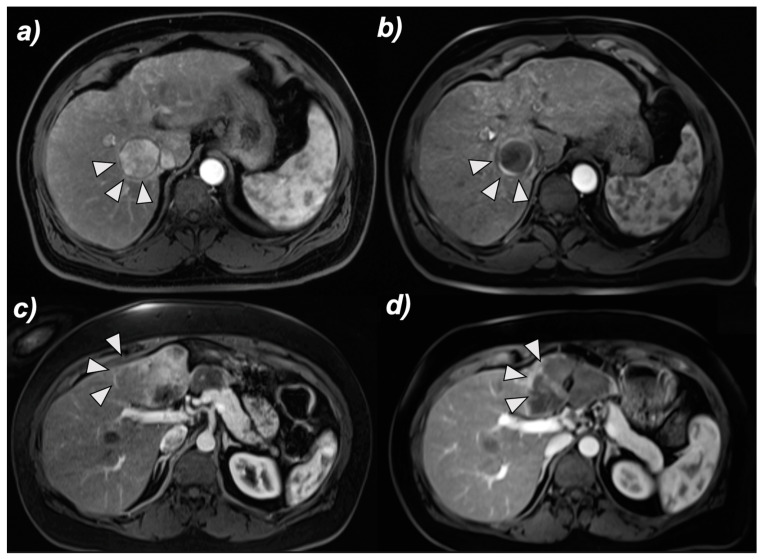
Imaging-based response of nodular and infiltrative HCC to TACE. Representative contrast-enhanced MR images of a nodular HCC treated (a) with DEB-TACE (upper row) and an infiltrative HCC (c) treated with cTACE (lower row), both showing good local tumor response on follow-up MRI after 4 weeks (b,d). Arrowheads indicate tumors on arterial-phase MRI scans. Hepatocellular carcinoma, HCC; DEB-TACE, drug-eluting beads TACE; cTACE, conventional transarterial chemoembolization.
